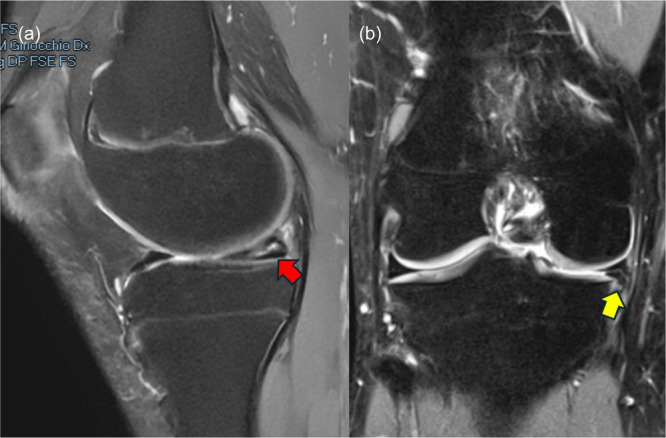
MRI features of degenerative meniscal lesions. (a) Horizontal cleavage tear. (b) Displaced flap tear.
